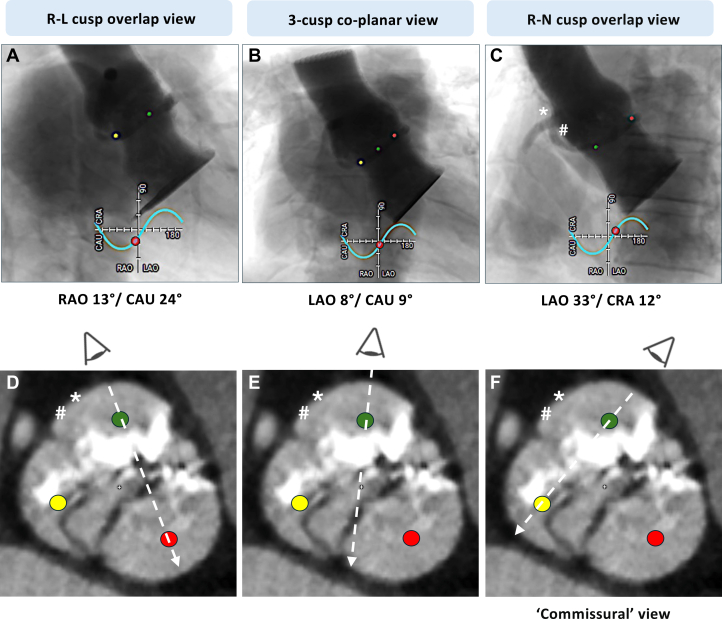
Computed Tomography–Derived Fluoroscopic Views (A to C) Three-dimensional CT reconstructed views. (D to F) Two-dimensional CT axial planes a few millimeters above the aortic annulus level. (A) and (D) show the R-L cusp overlap view. (B) and (E) show the 3-cusp coplanar view. (C) and (F) show the R-N cusp overlap view, also called the “commissural view” in case of a type 1 bicuspid aortic valve with R-N fusion. The yellow dot denotes the noncoronary cusp, the green dot denotes the right coronary cusp, and the red dot denotes the left coronary cusp. ∗ostium of the right coronary artery; #ostium of the left coronary artery. CAU = caudal; CRA = cranial; CT = computed tomography; LAO = left anterior oblique; RAO = right anterior oblique; R-L = right coronary–left coronary; R-N = right coronary–noncoronary.
